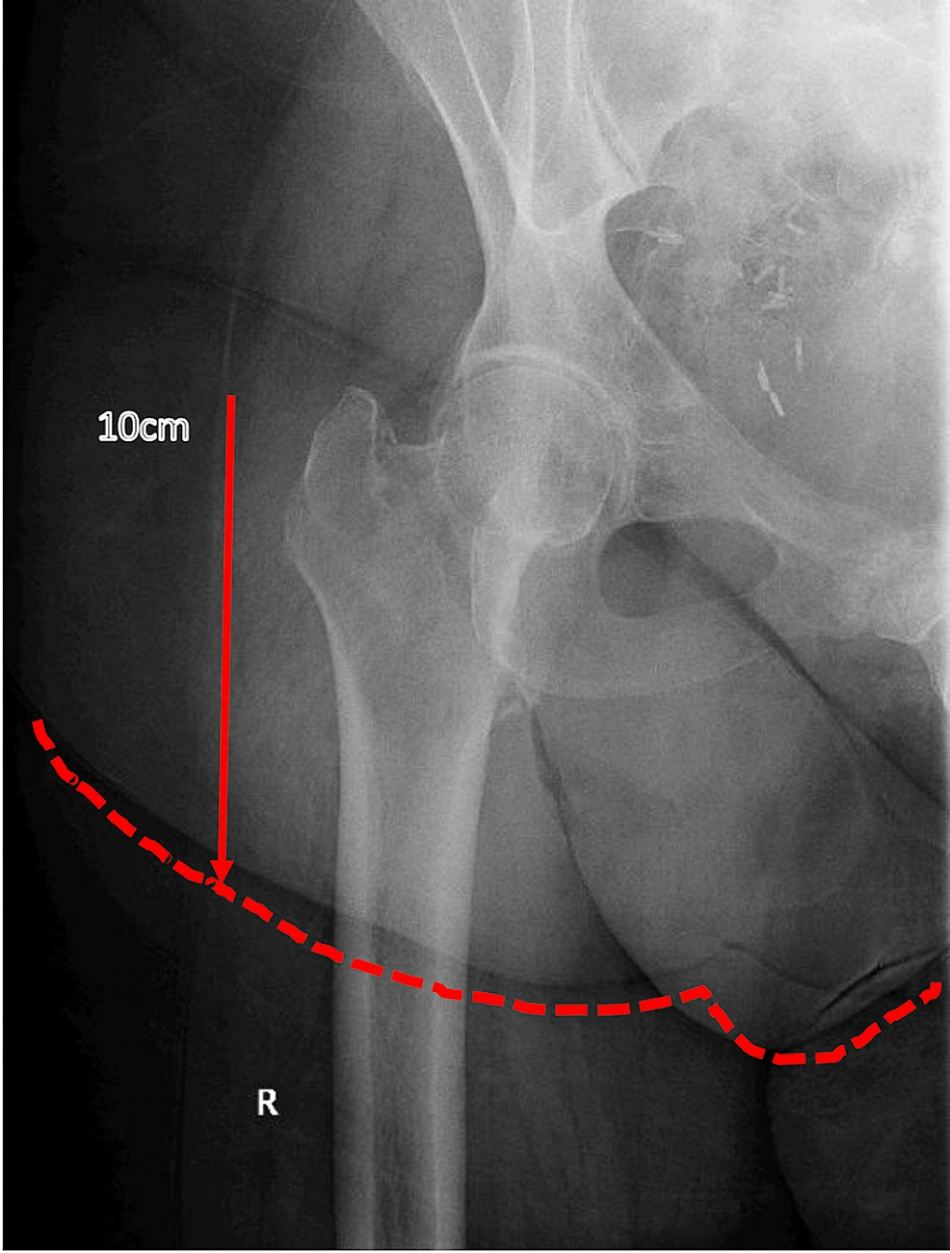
Initial Imaging Anteroposterior radiograph of the right hip demonstrating OTA/AO type 31A1.2 intertrochanteric fracture. The abdominal panniculus fold, extending 10cm distal to the tip of the greater trochanter and outlined in red, is overlying the anterior and lateral aspect of the right hip joint. OTA/AO: Orthopaedic Trauma Association/Arbeitsgemeinschaft für Osteosynthesefragen
